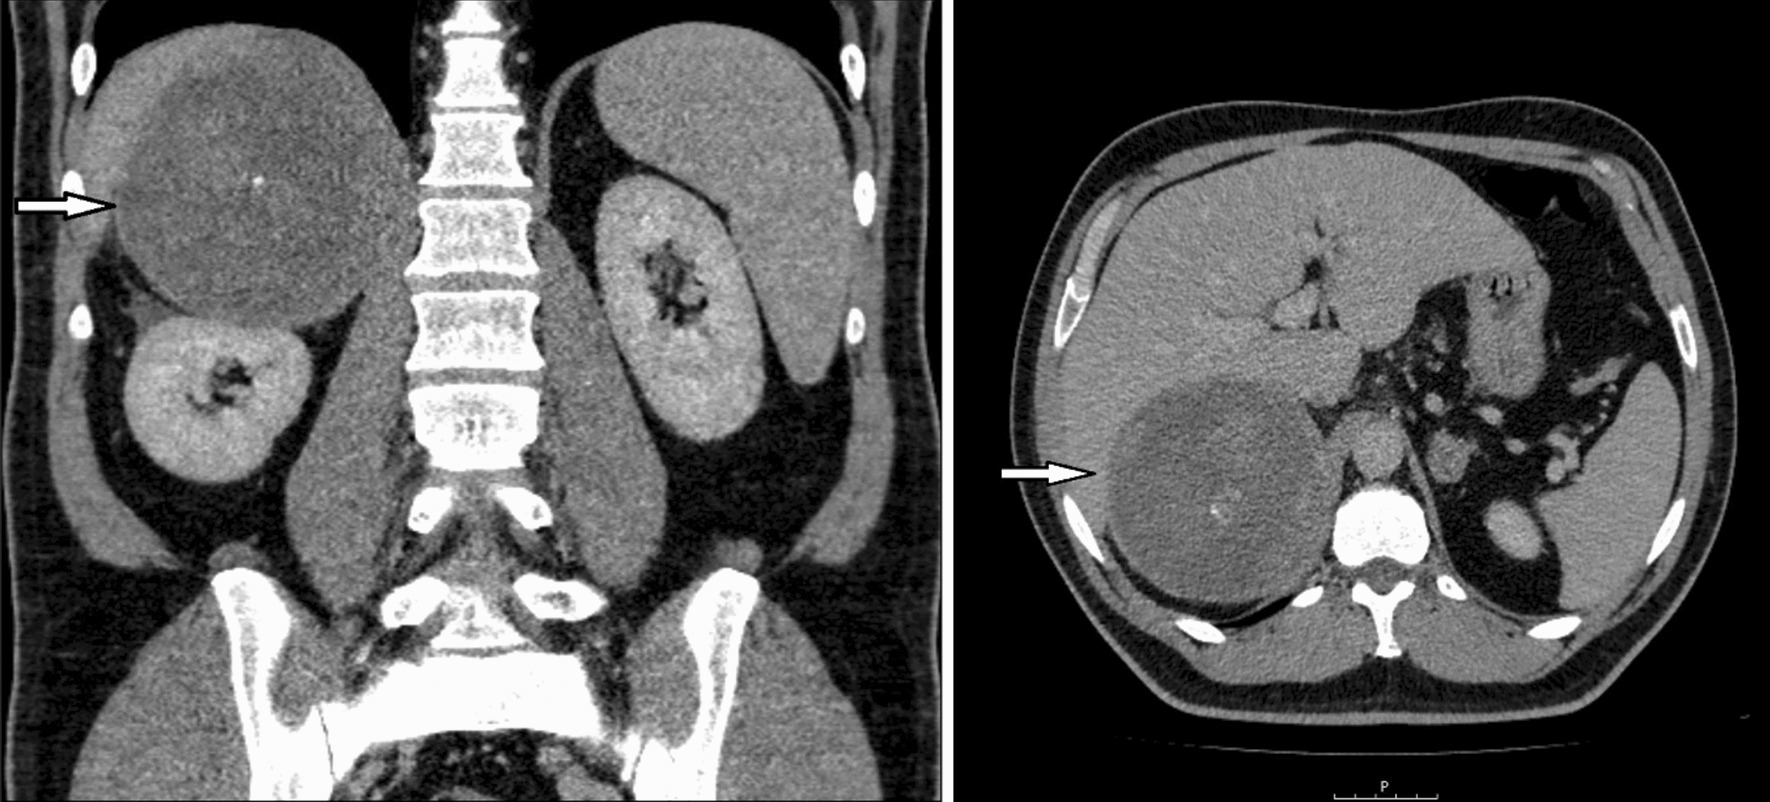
Contrast-enhanced computed tomography scan showing a heterogeneous mass (11.5 × 10.5 cm, marked by arrows) in the right adrenal gland with a central calcification
